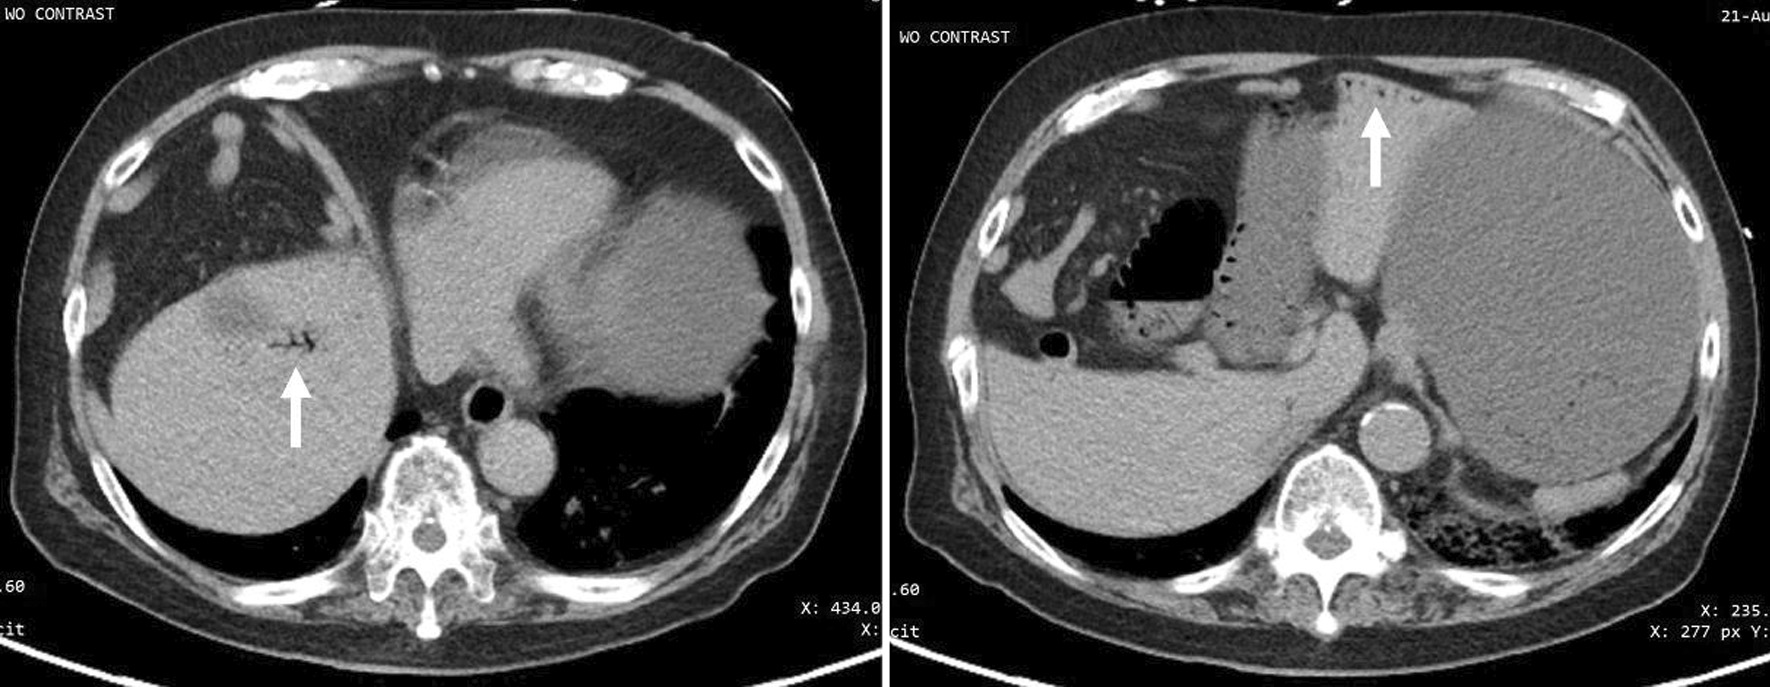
Portal venous gas. CT of the abdomen without contrast. Branching lucencies in the peripheral anti-dependent liver (solid arrows) compatible with portal venous gas
